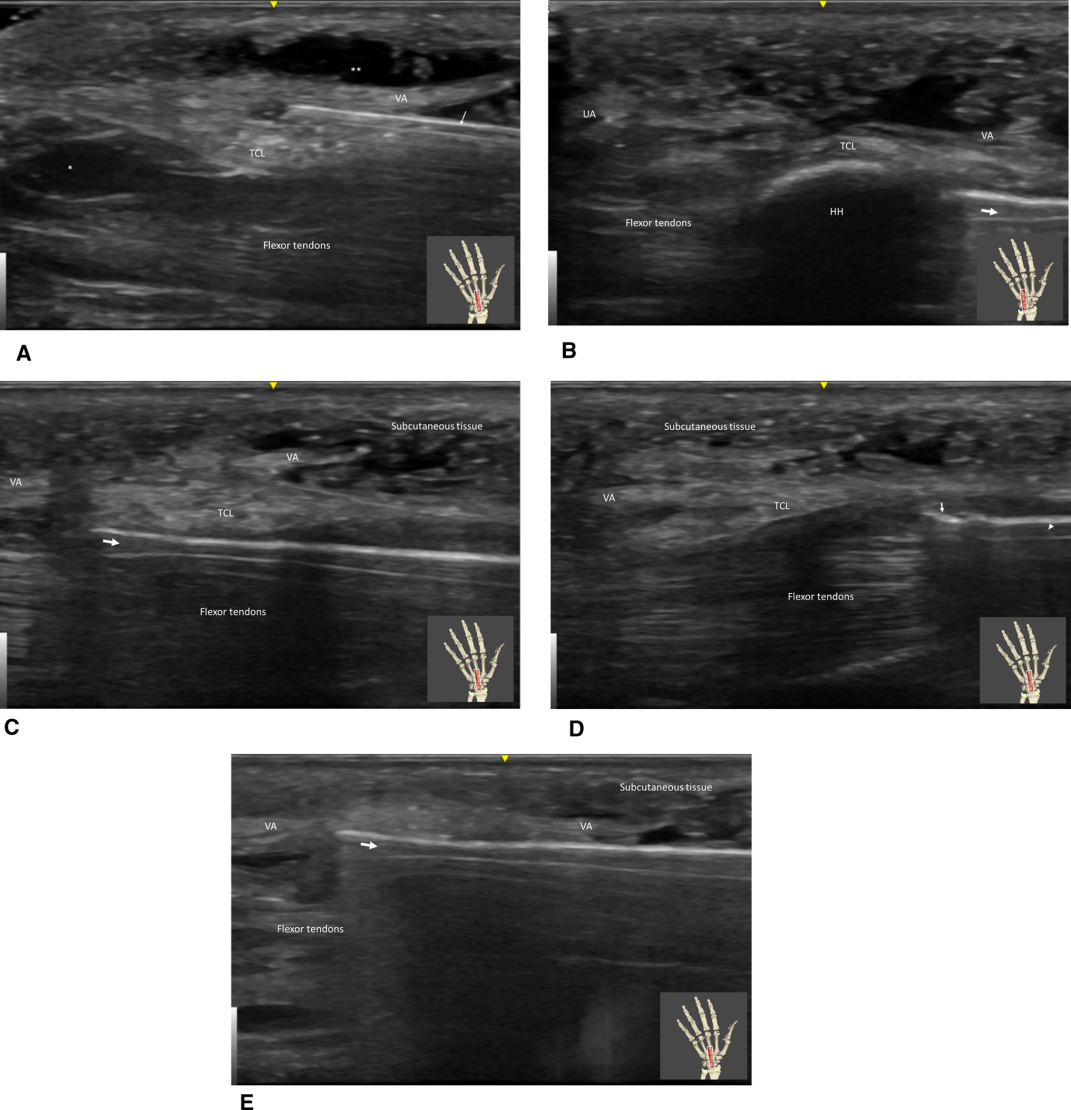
A Carpal tunnel in long axis view after xylocaïn injection under (*) the transverse carpal ligament (TCL) and over () the volar aponeurosis (VA). Contrast enhanced by local anesthesia injection improve the visualisation of the ligament. The puncture needle is locate between the TCL and the volar aponeurosis (arrow). B Carpal tunnel in long axis view passing at the level of the hamate hook (HH). The 1.5 mm probe (arrow) introduced through the puncture point is use to palpate anatomical structures like hamate hook (HH) to devine the best release trajectory. Note the ulnar artery (UA) crossing to become the superficial volar arch and the volar aponeurosis just above the transverse carpal ligament (TCL). C Carpal tunnel in long axis view passing just radial to the level of the hamate hook. The 1.5 mm probe (arrow) introduced through the puncture point is use to define the best release trajectory (along the safe zone). The 1.5 mm probe is moved from deep to superficial but remain in the carpal tunnel, stumbling over the transverse carpal ligament (TCL). Note the volar aponeurosis (VA) standing between the TCL and the subcutaneous tissue. D Carpal tunnel in long axis view passing just radial to the level of the hamate hook. The CT-SI Sonoinstrument (arrow head) is introduced through the puncture point and cut the transverse carpal ligament (TCL) along the safe zone. Note the cutting part of the instrument higlighted by the hyperechoïc line at its tip (arrow). Note the volar aponeurosis (VA) standing between the TCL and the subcutaneous tissue distally. E Carpal tunnel in long axis view passing just radial to the level of the hamate hook. After the release the 1.5 mm probe (arrow) introduced through the puncture point to palpate the potential ligament remnant. The 1.5 mm probe is moved from deep to superficial and is able to pass softly from the carpal tunnel, to the deep aspect of the volar aponeurosis (VA) or to the subcutaneous tissue if complete release is achieved
